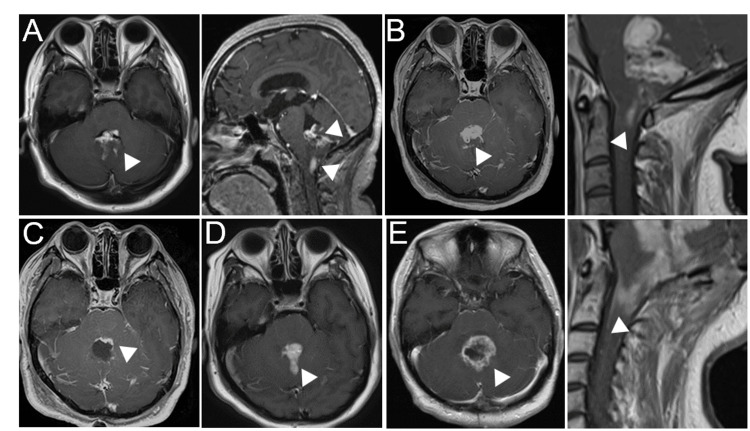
MRI images during the clinical course of Case 2. (A) MRI contrast-enhanced T1-weighted images (left; head axial image and right; head and cervical sagittal image) at initial onset. (B) MRI images (left; head axial image and right; cervical sagittal image) at regrowth of the tumor. (C) MRI image after tumor removal. (D) MRI image after two months of tumor removal. (E) MRI images (left; head axial image and right; cervical sagittal image) after radiation therapy.
